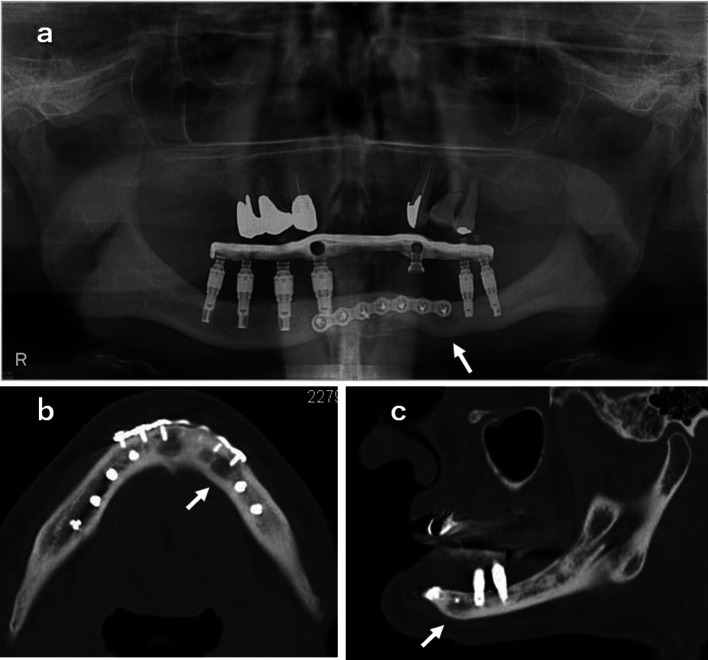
Bone fusion was observed 1 year after superstructure attachment at fracture site ( →) a panoramic radiograph, b horizontal CT image and c sagittal CT image
